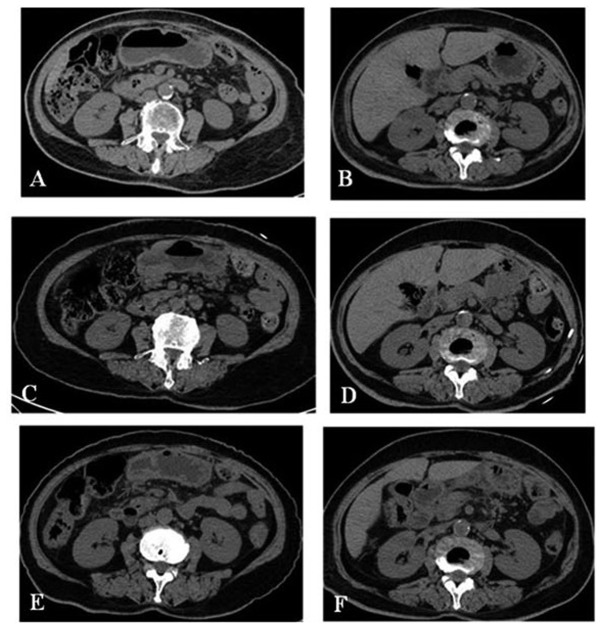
(A) Stomach CT following surgery. (B) CT demonstrated no lymph node metastasis in the abdominal cavity. (C) Stomach CT showed recurrence. (D) CT demonstrated lymph node metastasis in the abdominal cavity. (E) Stomach CT showed that the mass was reducing in size. (F) CT demonstrated that the abdominal cavity lymph node was reducing in size.
